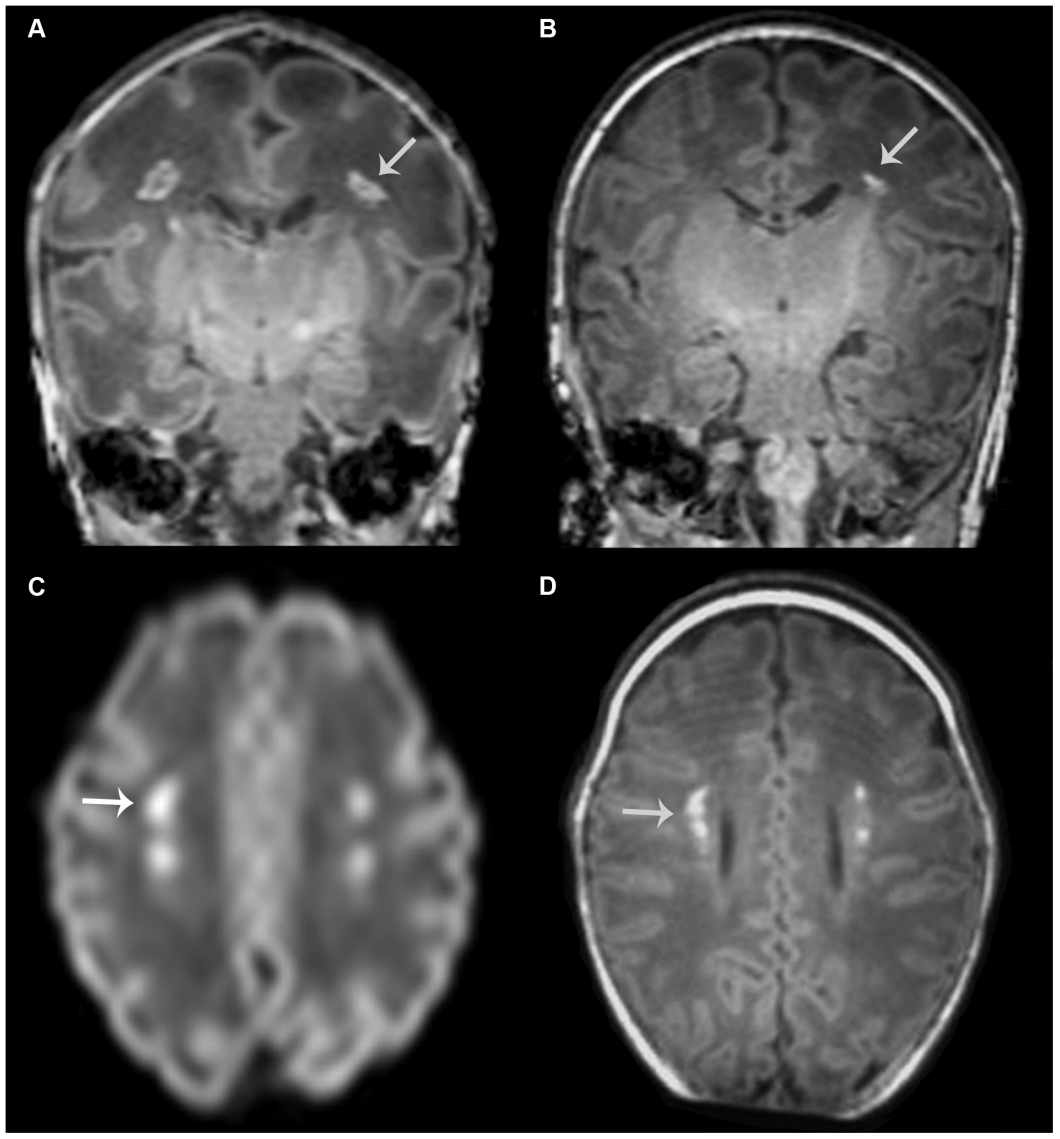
PWML in an infant without additional lesions who developed cerebral palsy. T1-weighted images at early (born at 31 weeks, postmenstrual age at scan 33 weeks, (A)) and term equivalent age (B) in an infant with lesions in a cluster pattern. Note that the lesions seem to be directly in the path of the corticospinal tracts (see arrow for an example). Lesions show high signal intensity, suggestive of restricted diffusion, on DWI of the early scan (C) and these are visible in the same location on T1-weighted imaging at term equivalent age (D). No signal intensity changes were seen on SWI on either scan and no additional lesions were identified. At term equivalent age, the posterior limb of the internal capsule appears to be less well developed on the right side. This infant subsequently developed a mild asymmetric bilateral spastic cerebral palsy, with her left leg being most severely affected.
